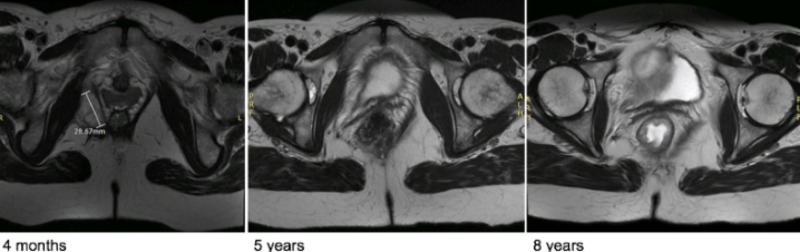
Pelvic MRI Postoperative evolution
